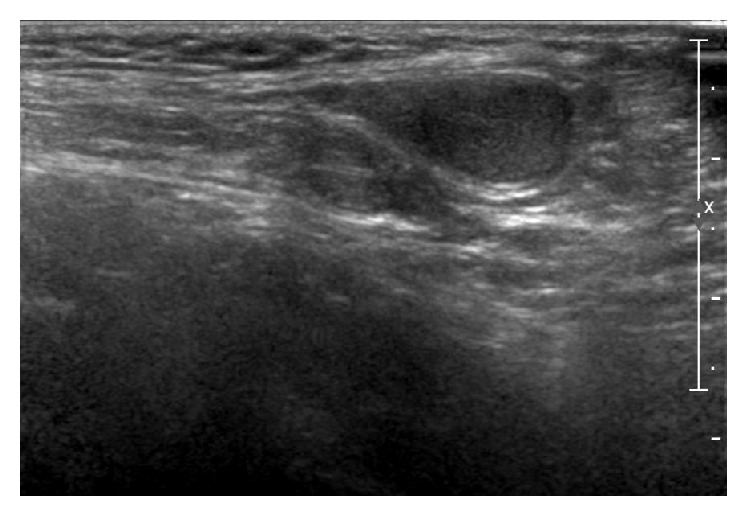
Left epididymis and testis with no pathological signs seen in scrotal ultrasonography.
